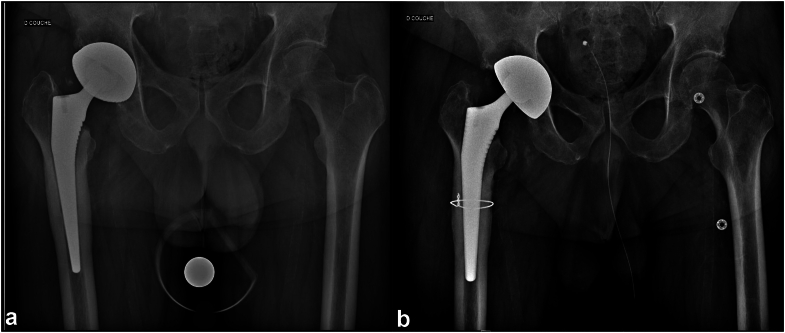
Suspended limited femorotomy. (a) Preoperative radiograph of a patient with right prosthetic hip infection. (b) Postoperative radiograph of a stem extraction with limited suspended femorotomy and reimplantation of a standard stem with 1 wire.
